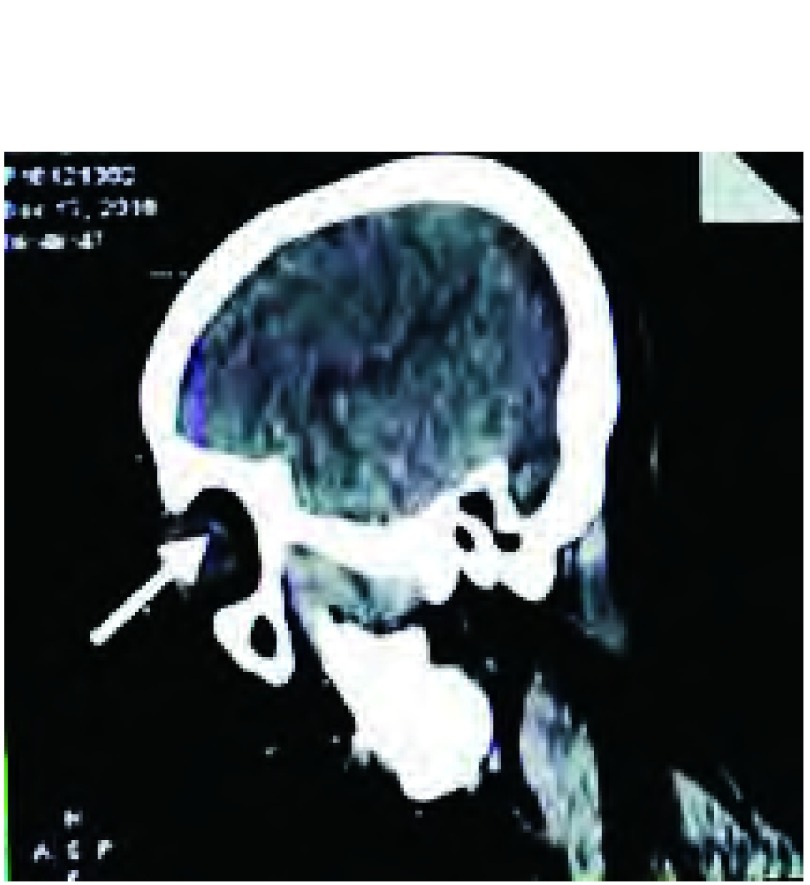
患者发病初期PET-CT检查显示左侧眼底局限性增厚，代谢不高。 At the onset of the disease, PET-CT showed a Localized thickening of the left fundus with low metabolism.
